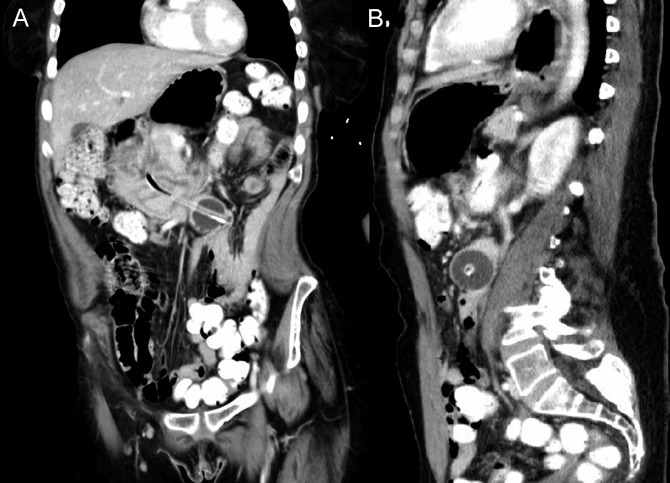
(A) Coronal and (B) sagittal abdominal computed tomography demonstrating retrograde jejunoduodenal intussusception with balloon migration into the second portion of the duodenum.
